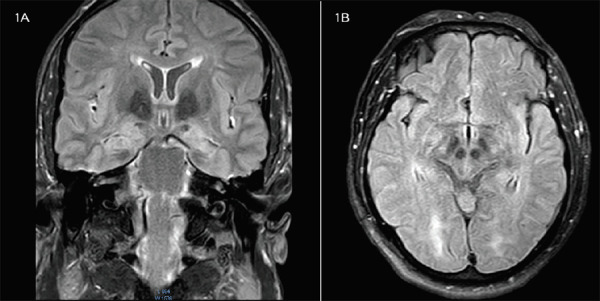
A, B. MRI showing increased FLAIR intensity signal in the bilateral hippocampus with atrophy of the left hippocampus and temporal lobes and insular cortex (A) Coronal view (B) Axial view.
